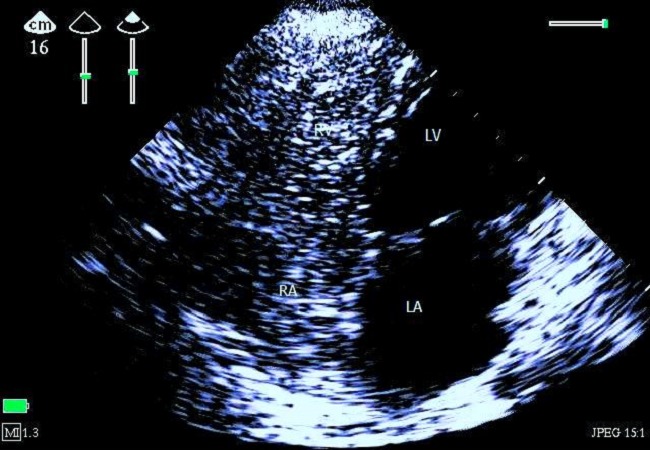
Apical four-chamber 2D echocardiography centered on the right cavities showing massive gas bubbles, image taken 50 seconds after the onset of clinical signs of embolism. (LA: left atrium, LV: left ventricle, RV: right ventricle, RA: right atrium)
